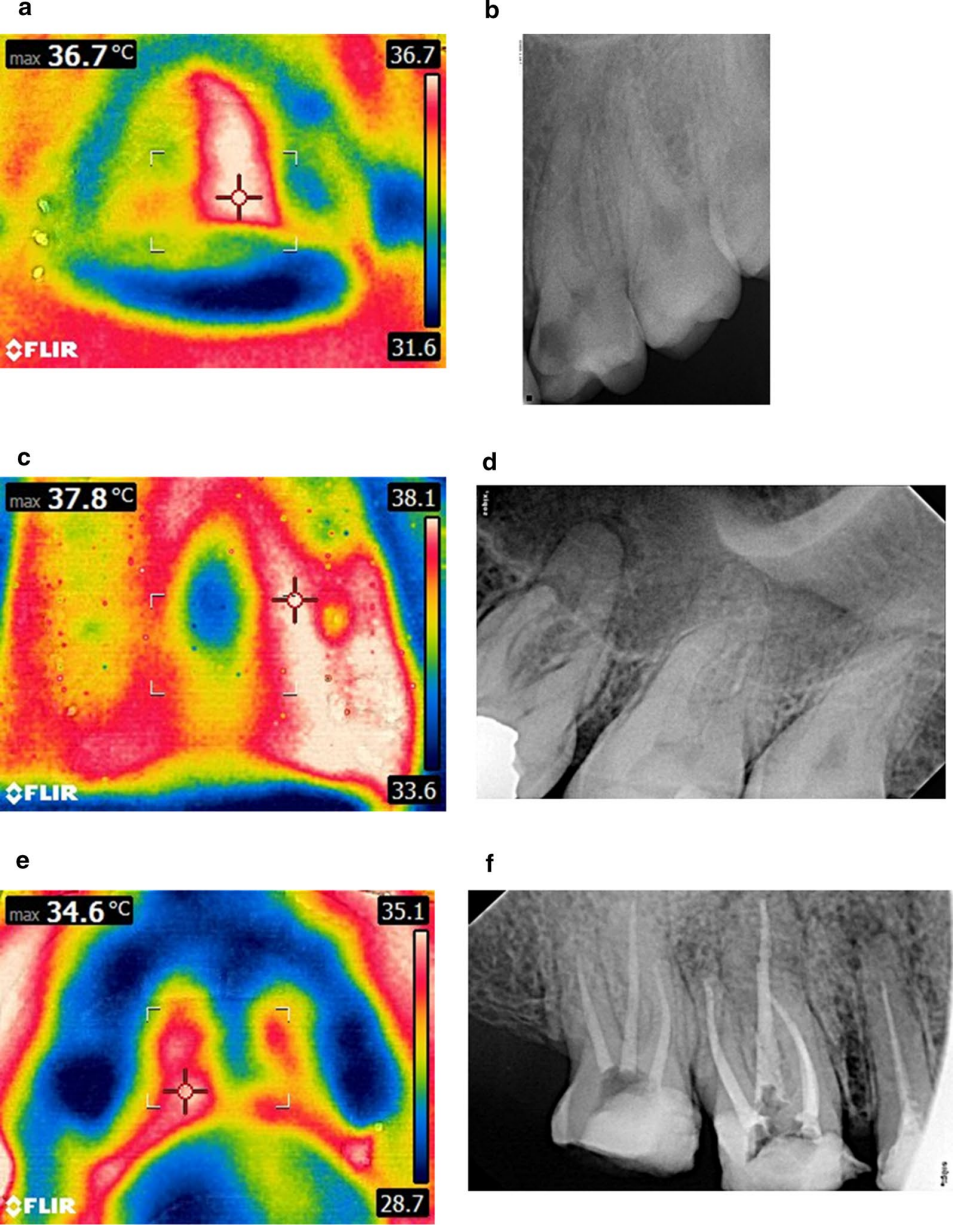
a Thermal image for AAP related to the upper left first molar. The heat detector is pointing to the highest temperature (36.7 °C). b Periapical radiograph showing widening of the lamina dura around the mesial root of the same tooth. c Thermal image for AA related to the upper left first molar with the highest temperature (37.8 °C) detected using Flir Tools software. d Periapical radiograph showing widening of the lamina dura around the roots of the same tooth with radiolucency related to the apex of the roots. e Thermal image for CA related to the upper right second premolar. f Periapical radiograph showing radiolucency surrounding the apex of the upper right second premolar. A sinus tract related to the same tooth was detected on clinical examination
